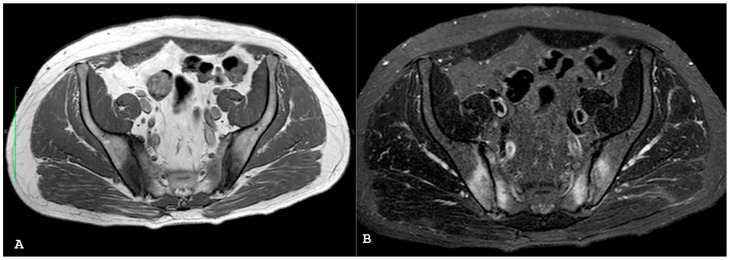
Axial T1-weighted (A) and axial short tau inversion recovery (B) magnetic resonance imaging sequences show bilateral periarticular bone marrow edema consistent with active sacroiliitis associated without bony irregularity.
